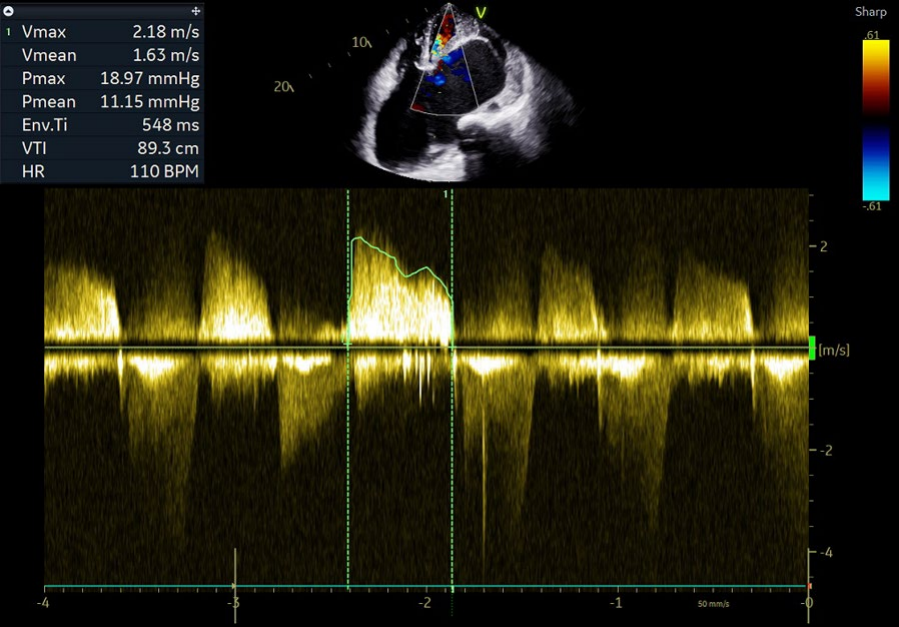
Two-dimensional echocardiographic assessment of severe mitral stenosis gradient and atrial fibrillation
